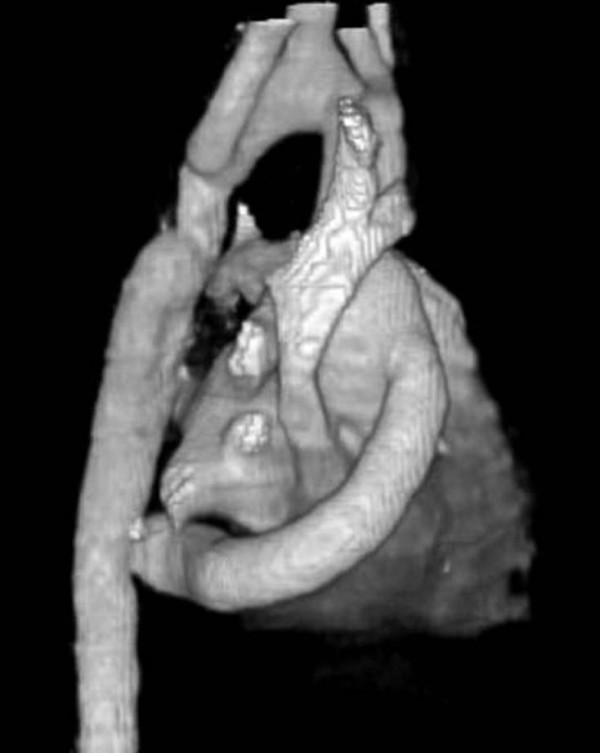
3-D reconstruction of postoperative CT scan showing the extra-anatomic bypass graft.
